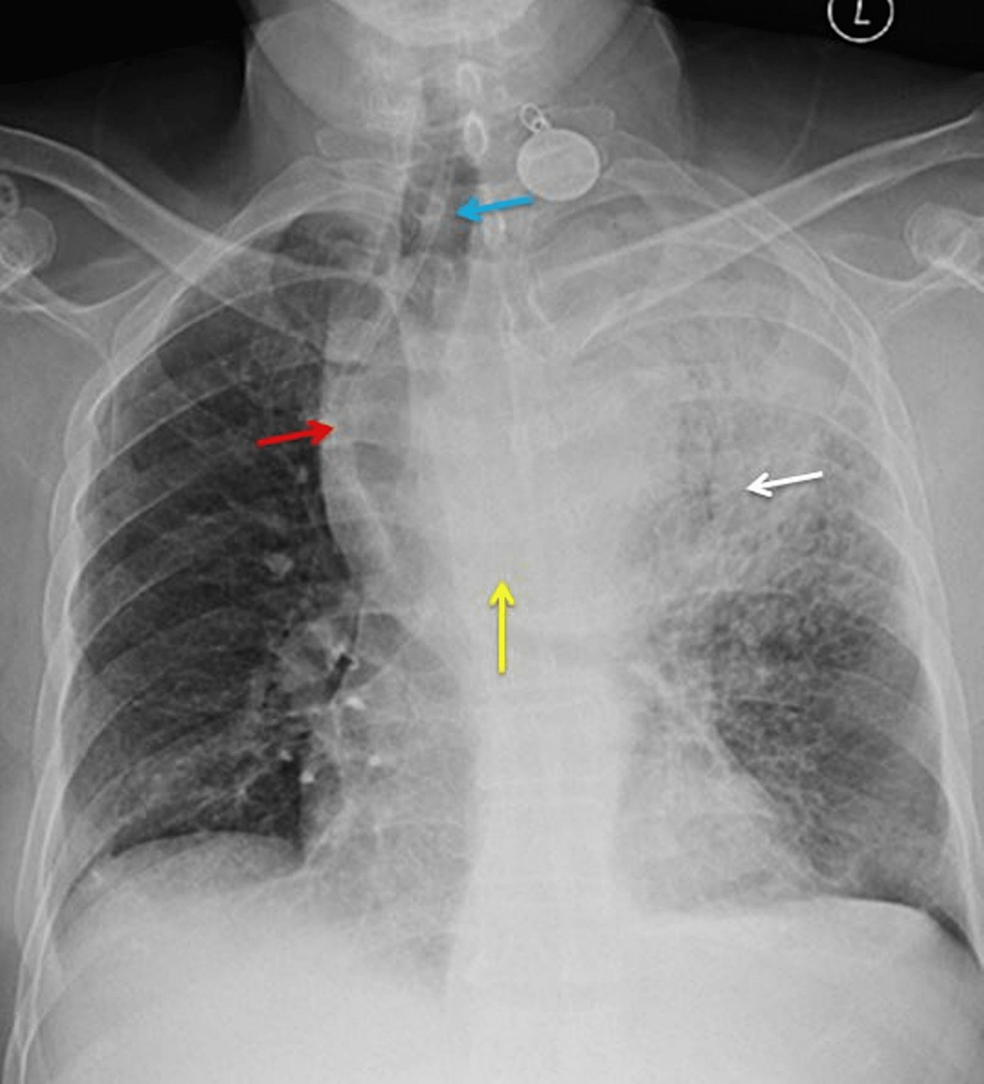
Chest X-ray showing haziness in the left lung (white arrow), aneurysm (yellow arrow, right-side shift of the trachea (blue arrow), widened mediastinum, and right-sided shift of the esophagus containing Ryle’s tube (red arrow)
